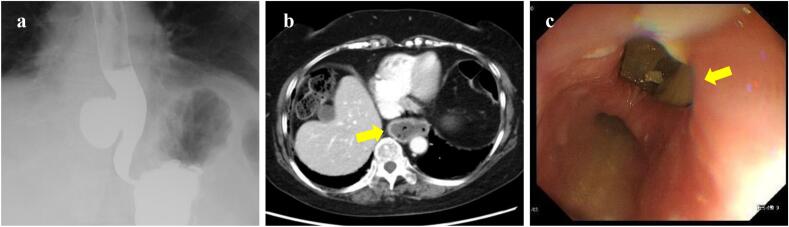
Preoperative findings. a. Contrast esophagography shows a 50-mm epiphrenic diverticulum on the right wall of the lower esophagus. b. Contrast-enhanced computed tomography shows a diverticulum (yellow arrow) at the lower mediastinum. c. Upper endoscopy shows the orifice of the diverticulum (yellow arrow).
